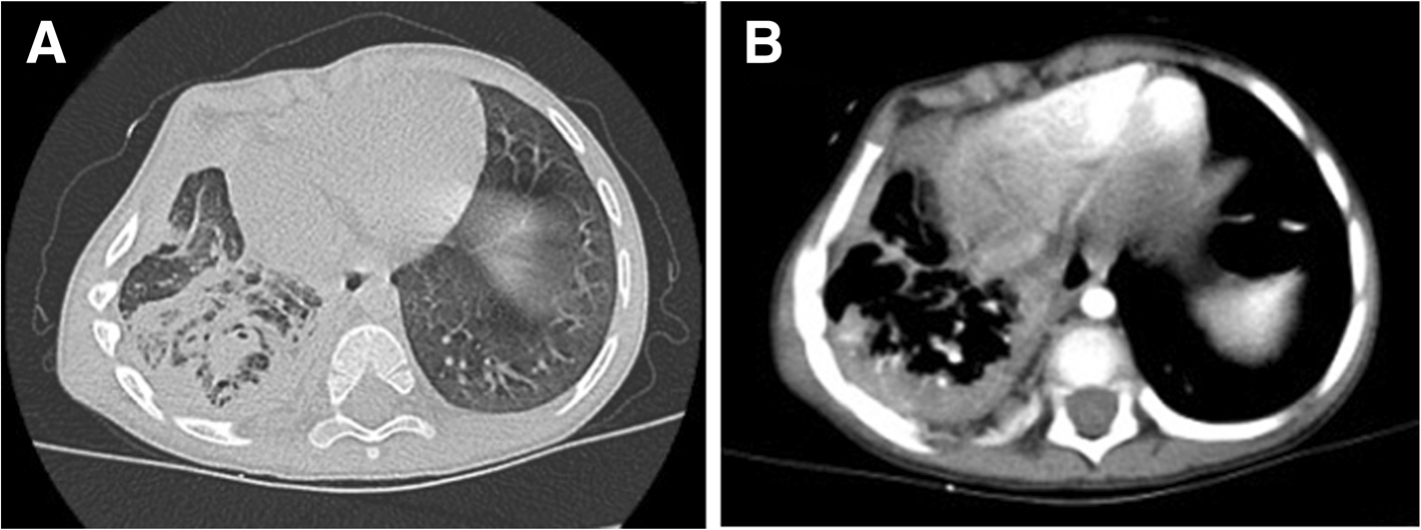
Lung CT showing the presence of 2a-b reduced right lung volume, diffuse high-density opacities with patchy shadowing and stripes, many small areas of calcification and cavitation in the lower lobe of right lung, irregular soft tissue of mixed density in the right inferior mediastinum, and calcification in the thickened pleura
